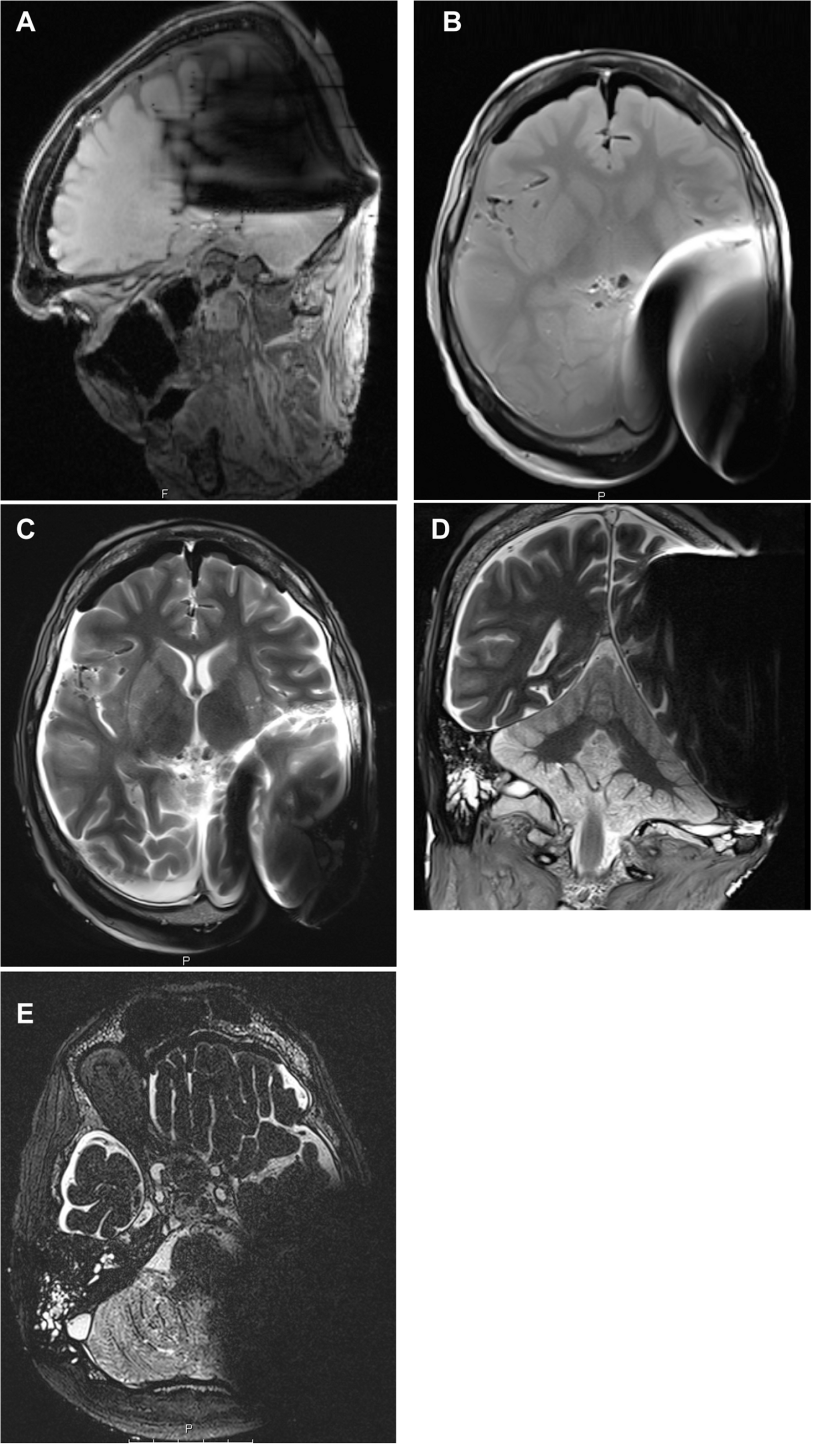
MR image series of one corpse head at 1.5T with magnet in situ. 2a: T1w MPR sagittal; 2b: T1w axial; 2c: T2w axial; 2d: T2w coronal;2e: CISS. The MR images show the maximum in diameter of the artefact in each sequence.
